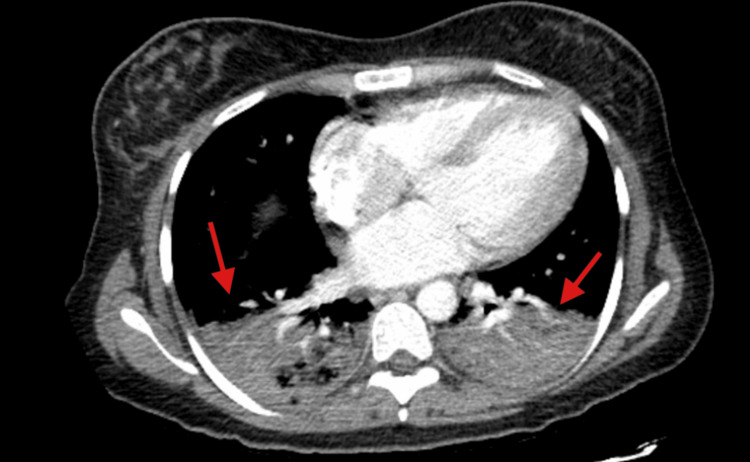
Computed tomography angiogram of chest in case 4 which was negative for pulmonary embolism but positive for bilateral lower zone consolidations (red arrows).
